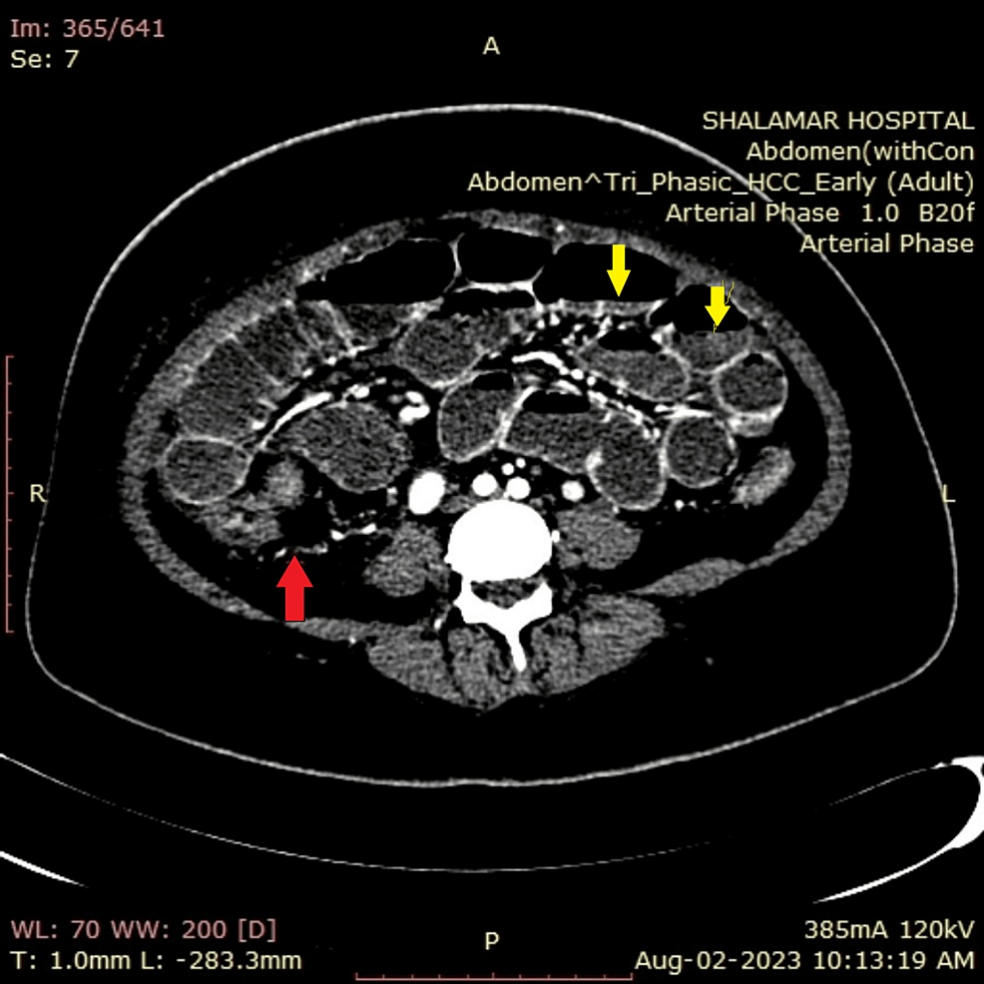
CT angiogram showing decreased vascular enhancement (red arrow) and air-fluid levels (yellow arrows).
